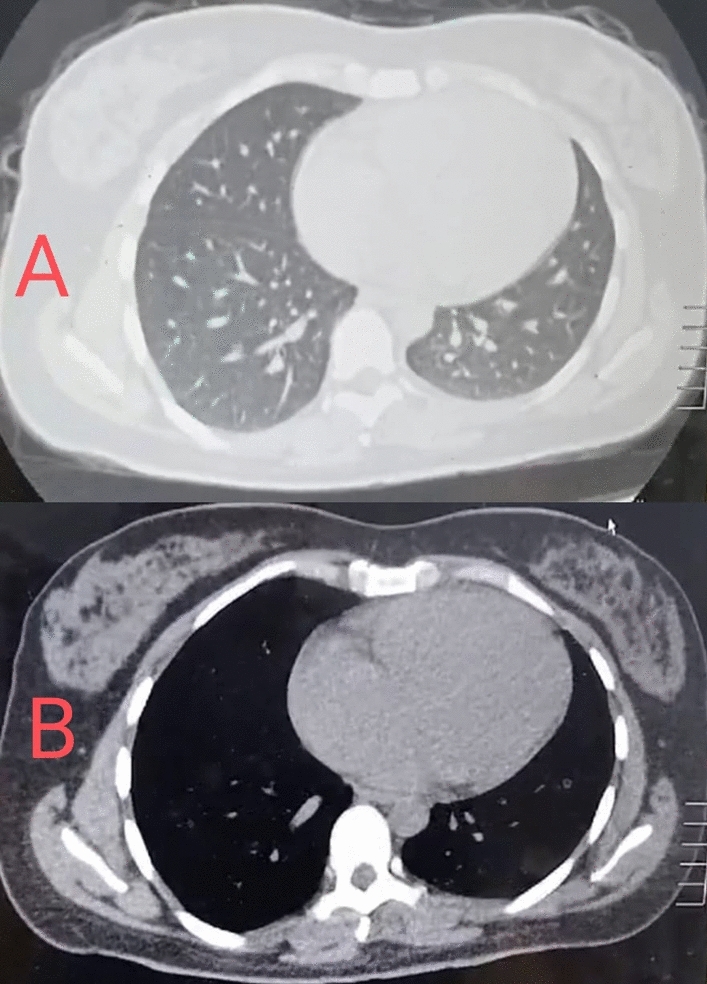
Axial CT chest: (A) Lung window of the CT chest with no signs of pneumonic or interstitial infiltration, (B) Mediastinal window suggestive of right side dilatation.
